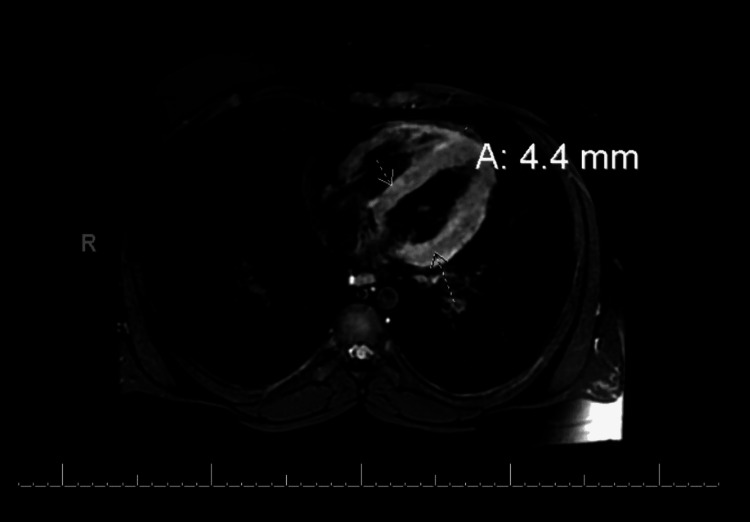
Cardiac MRI The image shows patchy mural delayed myocardial enhancement with relative sparing of the endocardium within the septum, and inferior and lateral walls at the cardiac base MRI: magnetic resonance imaging
